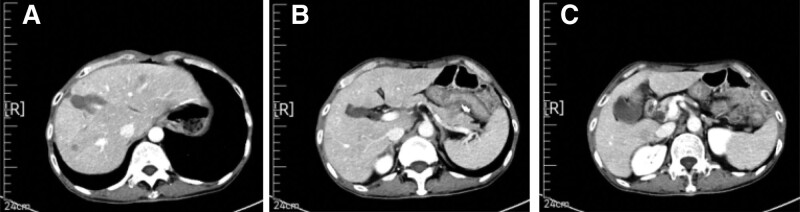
MR (2022-9-15) showed an increase in nodules in the right lobe of the liver compared to previous MR images on June 18, 2022; pancreatic tail nodule, considering metastasis.
